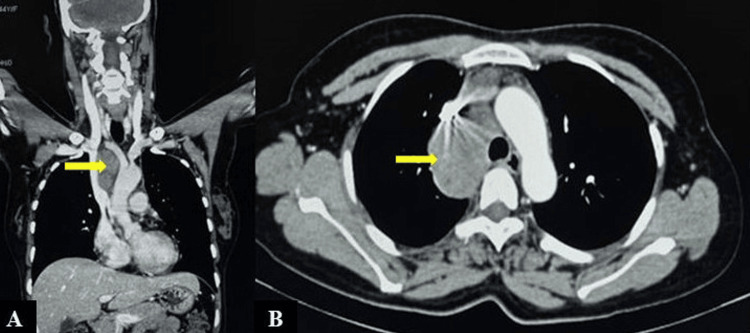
CT of neck, thorax and abdomen (A- Coronal view, B- Axial view) shows a mild homogenously enhancing mediastinal mass (6.6x5.4x4.0 cm) in right paratracheal region abutting the left distal brachio-cephalic vein and superior vena cava, shown by yellow arrows
